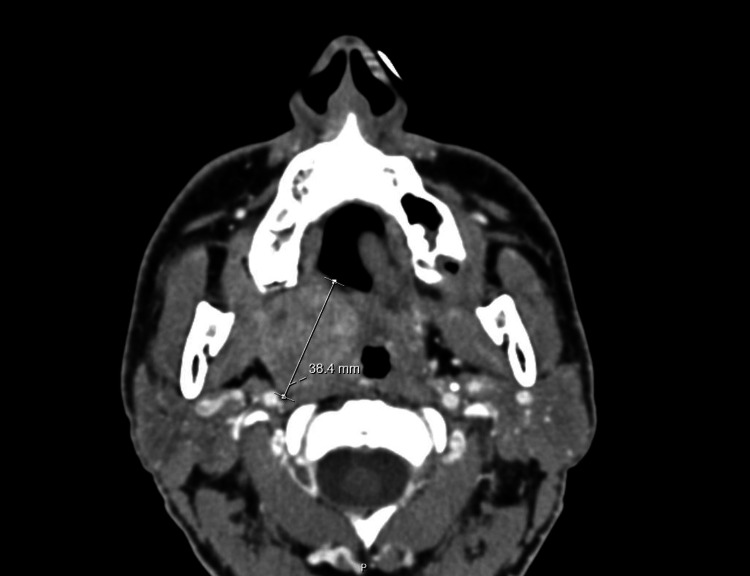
CT scan: transverse plane (mass with approximately 39 mm). CT, computed tomography
